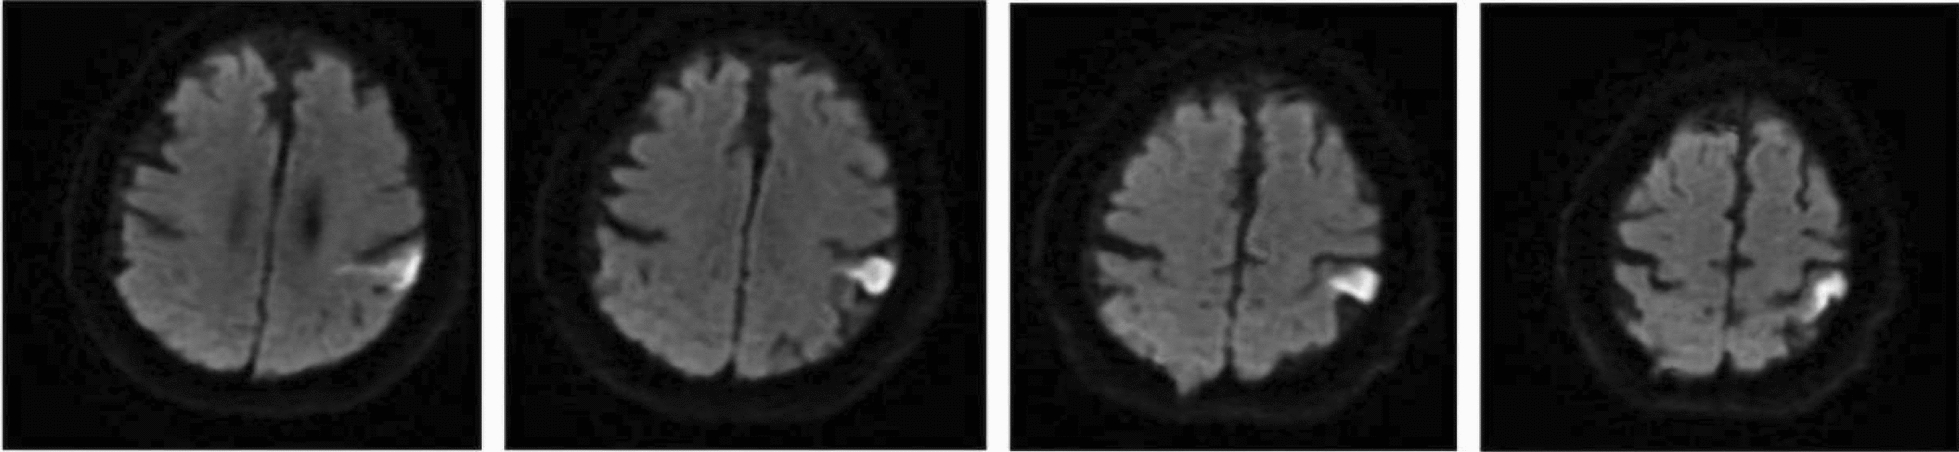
Diffusion-weighted MRI imaging of the patient's brain shows a left-sided parietal stroke [76]
